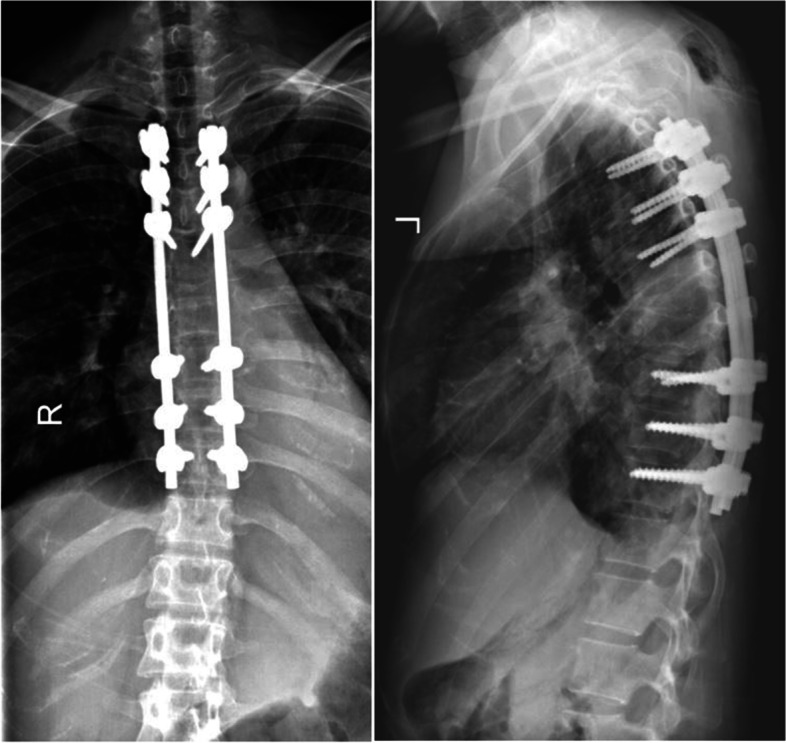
Post-operative radiograph showed the satify instrument position and spinal alignment
